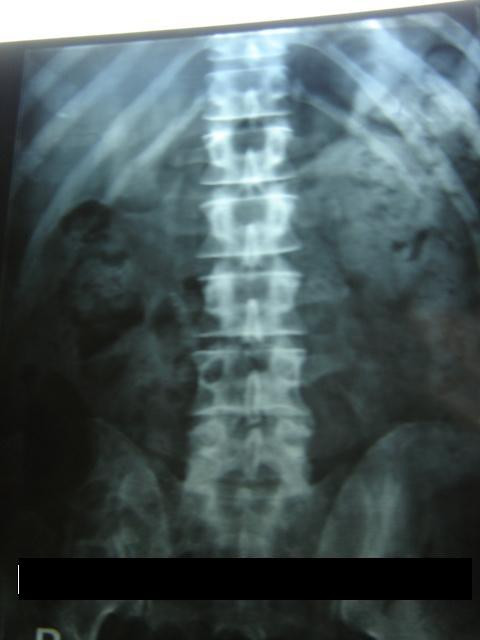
X-Ray of the paraspinal and pelvic region showing calcification.
